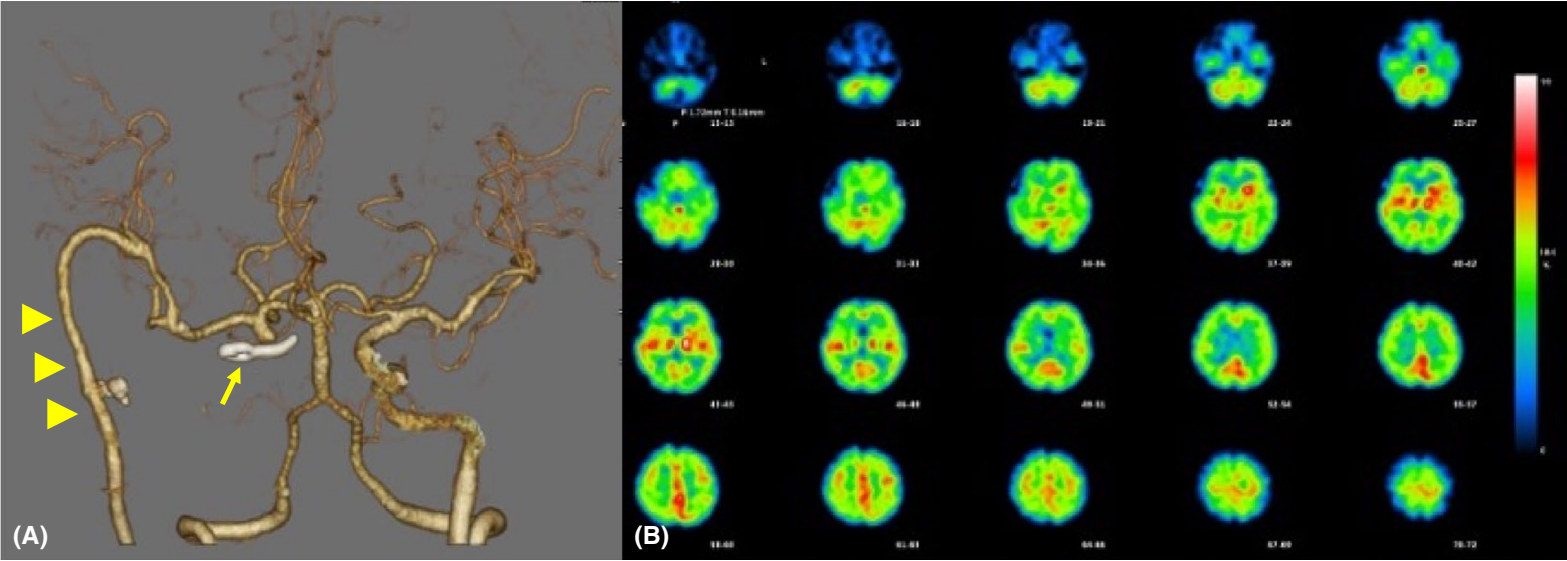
A. Postoperative CT angiography showing that revascularization is achieved by high‐flow bypass and peripheral arteries are sufficiently visualized. Arrowheads indicate radial artery graft as a high‐flow bypass. B. Postoperative single‐photon emission computed tomography image showing no decrease in cerebral blood flow
